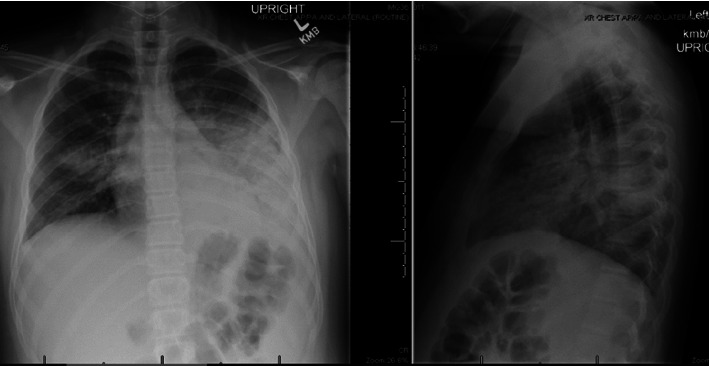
Anterior-posterior and lateral chest X-ray obtained at time of transfer from OSH for Case 2.
